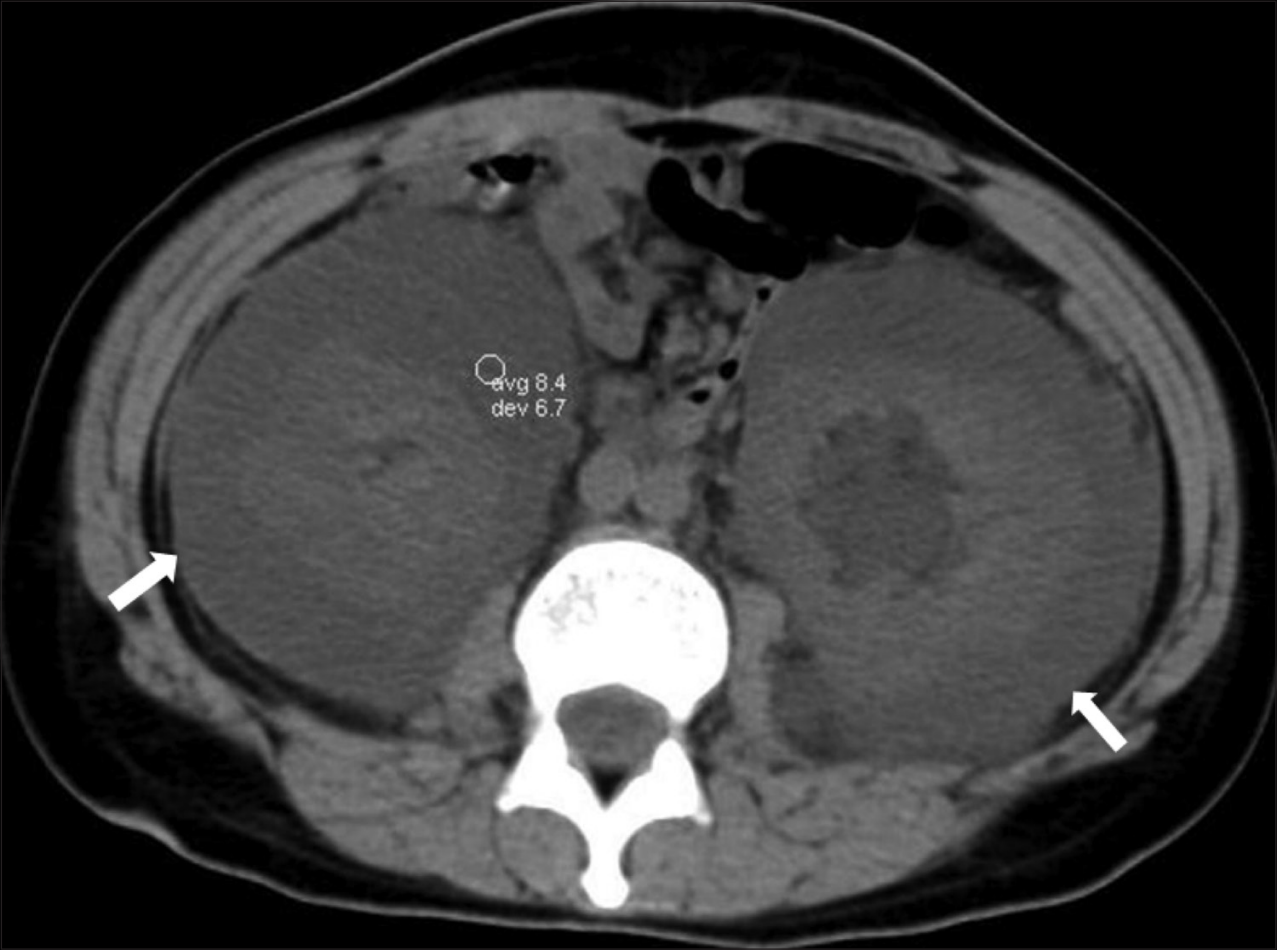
Non-enhanced, axial CT scan shows symmetric, hypodense perirenal collections (arrows) with an average Hounsfield value of 8
